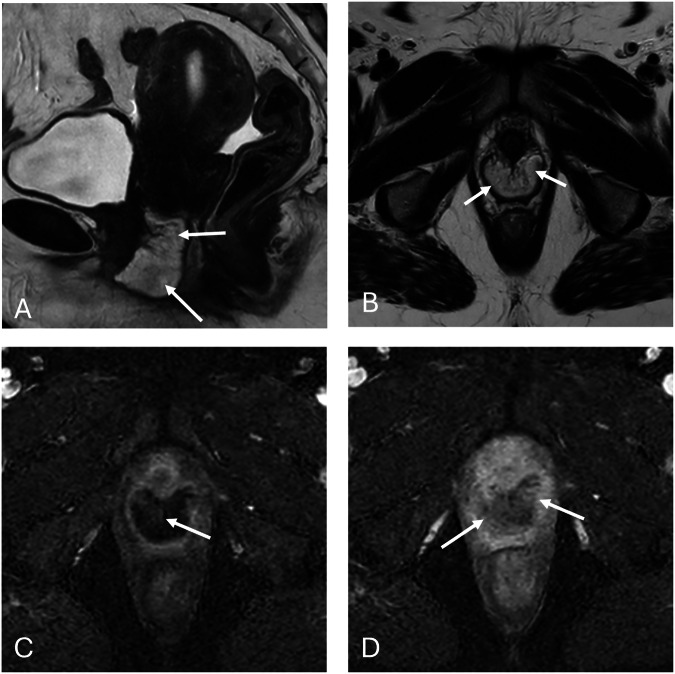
Vaginal gynaecological venous malformation (GVM) in a 48-year-old patient, G4P4, presenting with significant vaginal bleeding and a focal mass of the anterior vaginal wall identified several decades earlier during her first pregnancy. A Sagittal and B axial T2-W MR images show a well-defined thickening of the anterior vaginal wall, displaying T2-hyperintense signal (arrows). The rest of the genital tract appears normal. C Axial dynamic post-contrast T1-W fat-suppressed MR images at the arterial and D at the venous phase demonstrate slow, progressive enhancement of the lesion (arrows), without nidus or solid tissue component, suggestive of slow venous flow. Vaginal biopsy confirmed the diagnosis of GVM and identified a PIK3CA mutation
